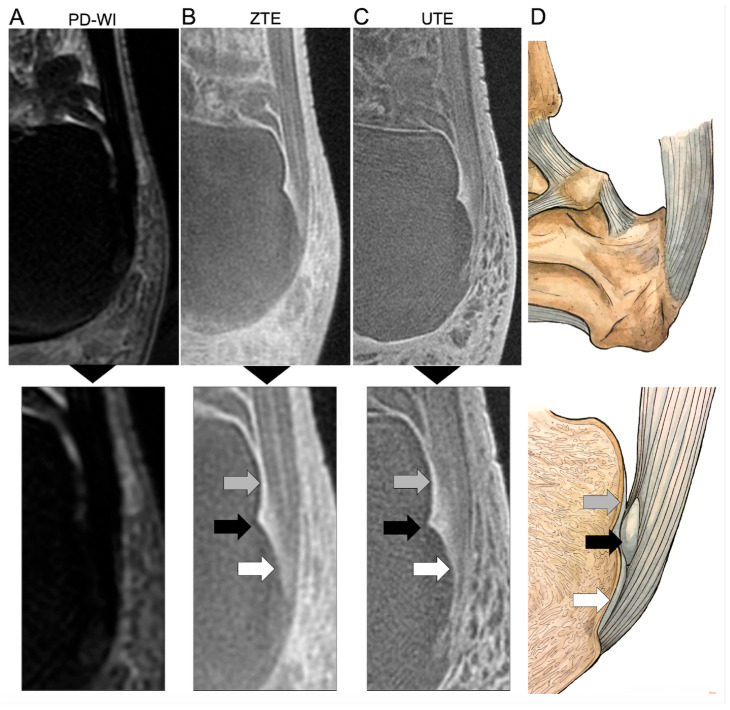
Assessment of the normal hindfoot and Achilles tendon ‘enthesis organ’ on proton density weighted imaging (PD-WI) ((A), upper panel) with magnification (lower panel), zero echo time imaging (ZTE) (B), and ultrashort echo time imaging (UTE) (C) MRI sequences and a corresponding representation of the real anatomy (D). The enthesis organ is made of three anterior fibrocartilages, all imperceptible with PD-WI, but demonstrating high signal intensity on UTE and ZTE sequences. The gray arrow shows the periost fibrocartilage (PCa) as a thin line at the posterior and superior surface of the superior tuberosity of the calcaneum. The black arrow shows the sesamoid fibrocartilage (SCa) as a ground-glass area within a notch at the middle of the posterior surface of the calcaneus. Lastly, the white arrow shows the enthesis fibrocartilage (ECa) at the interface of the tendon itself and the bone. The stripes on the Achilles tendon correspond to the endotenon (with high signal intensity on UTE and ZTE) and the tendon fascicle (with lower signal).
